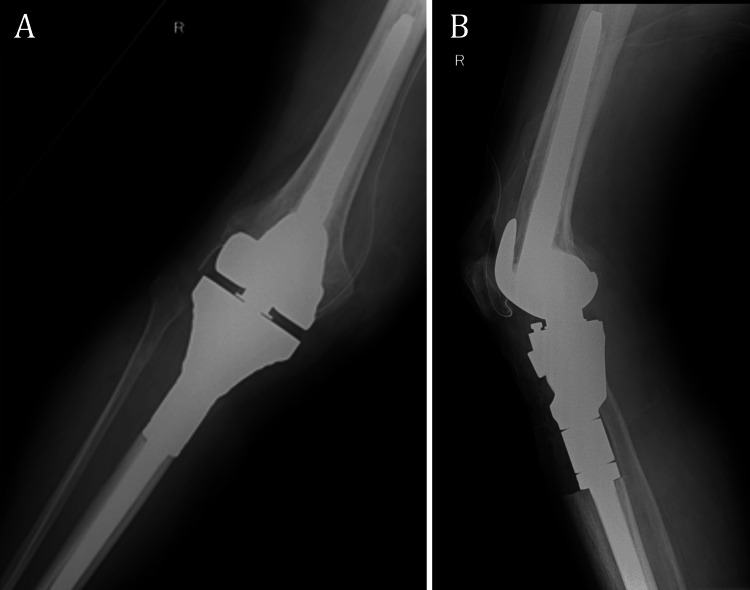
Postoperative radiographs of the right knee: (A) anteroposterior view; (B) lateral view.
